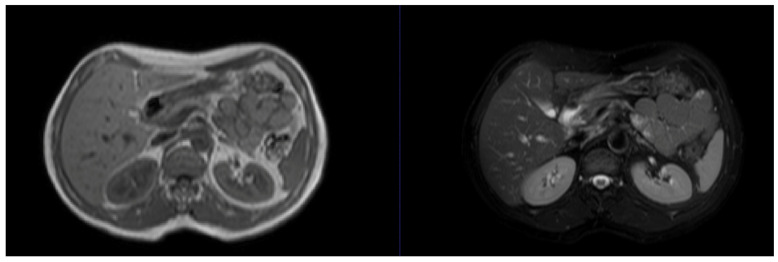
Example images from the CHAOS dataset [22]. CHAOS, Combined (CT-MR) Healthy Abdominal Organ Segmentation.
